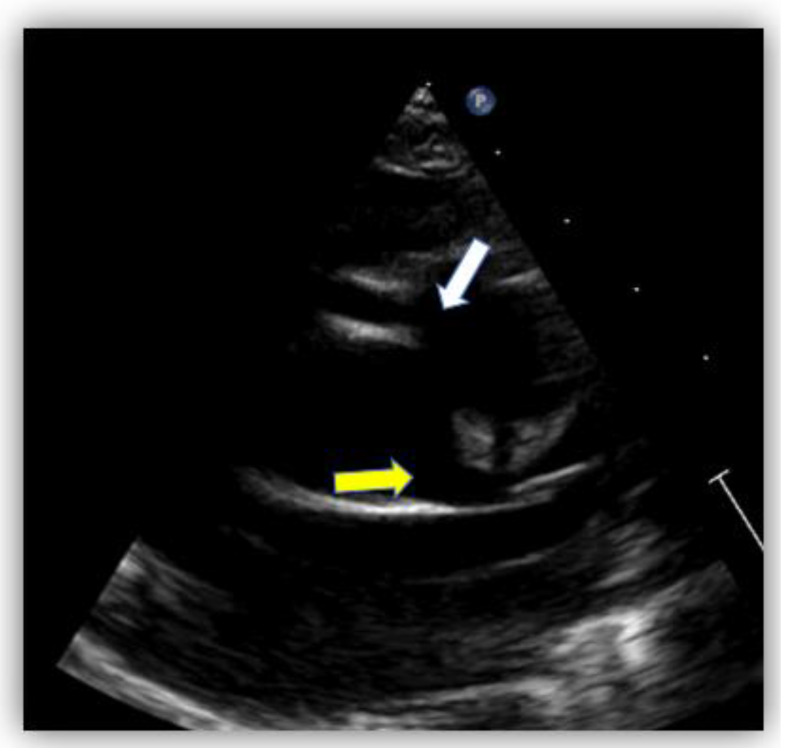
Echocardiography in the parasternal short-axis view. The white arrow shows the origin of the right coronary artery (RCA) from the pulmonary artery and the yellow arrow shows the normal origin of the left coronary artery (LCA) from the aorta.
